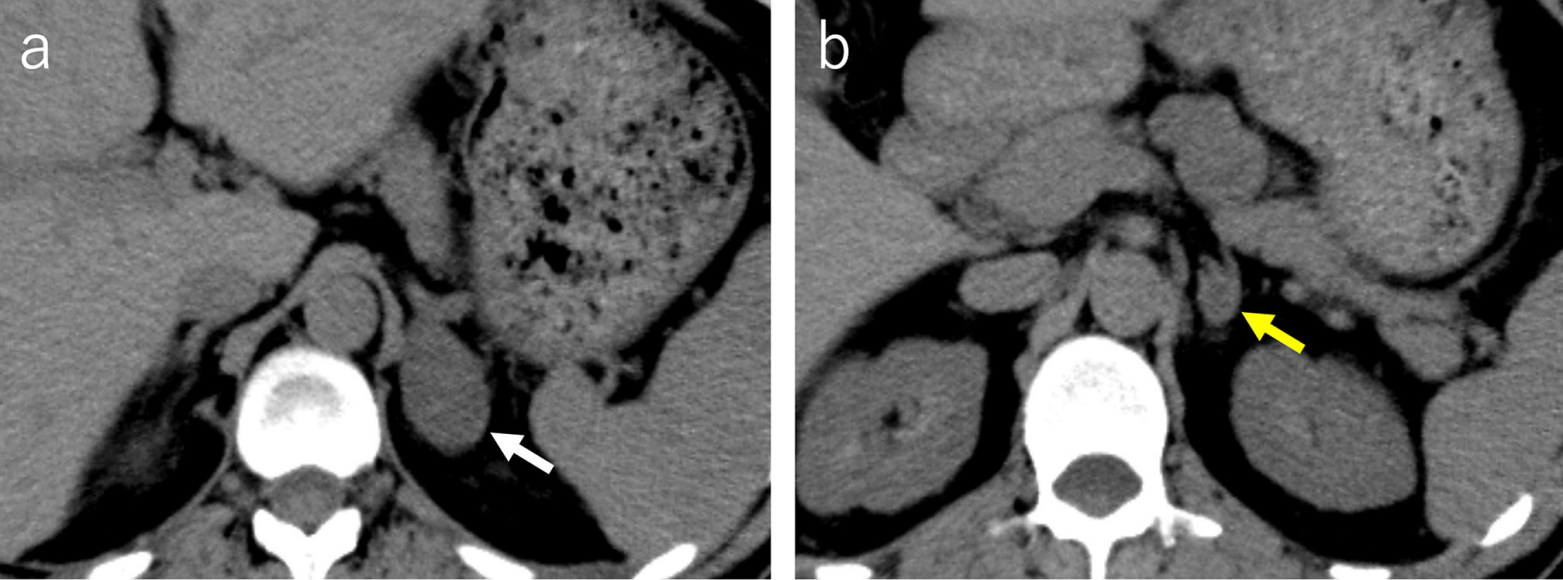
Plain computed tomography (CT) images. (a-, b) CT demonstrates the 6 cm cephalic lesion (white arrow) and the 1.5 cm caudal lesion (yellow arrow) in the left adrenal gland.
